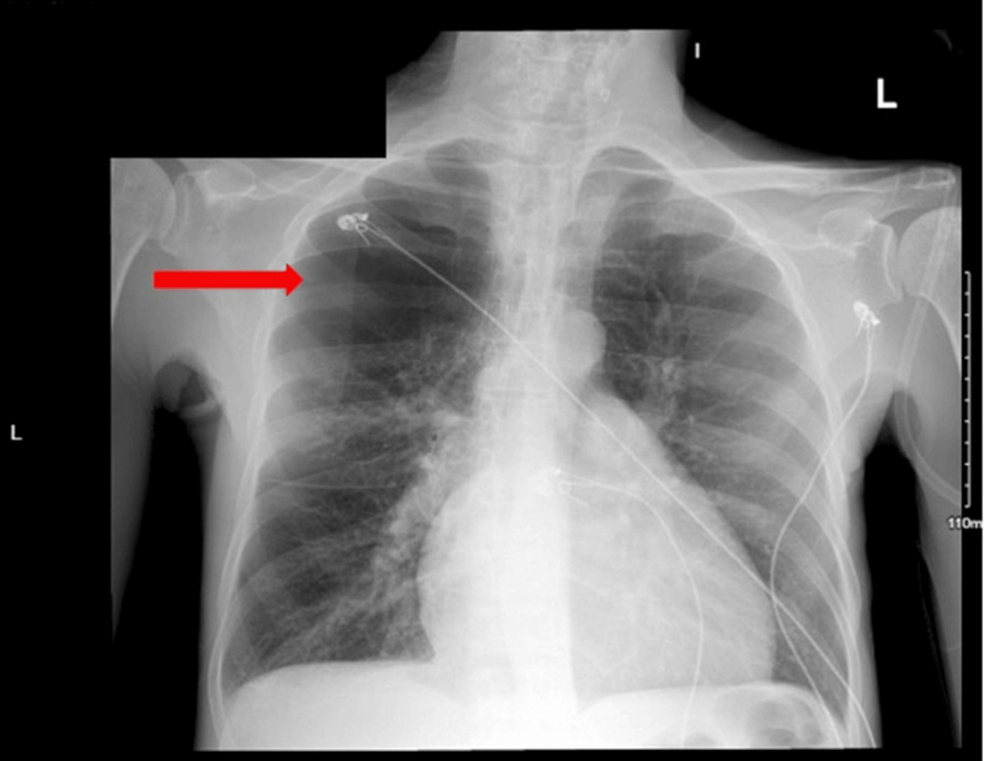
Chest X-ray of the patient showing a large right apical bulla (red arrow), prominence of the main pulmonary artery, and emphysematous changes in the lungs
